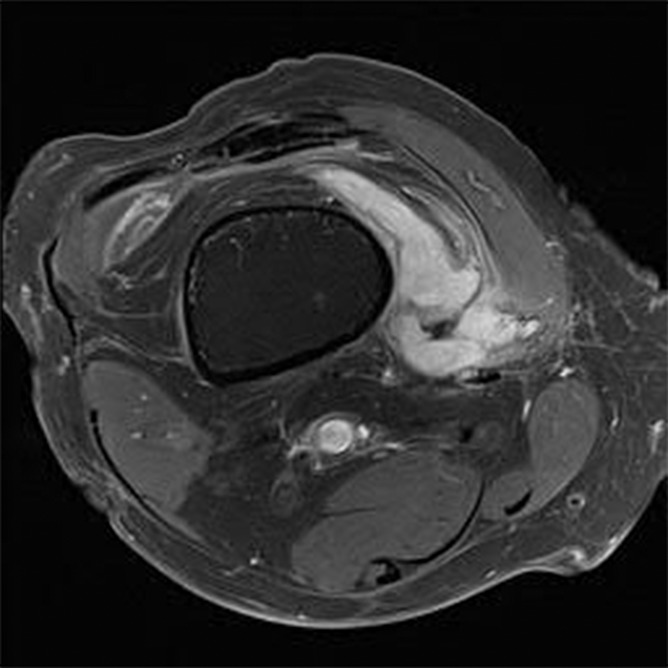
Axial T1-weighted MRI displaying peripheral nodular enhancement along the medial aspect of the distal femur likely reflecting hematoma measuring 13.4 × 4.6 × 3.0 cm. MRI, magnetic resonance imaging.
